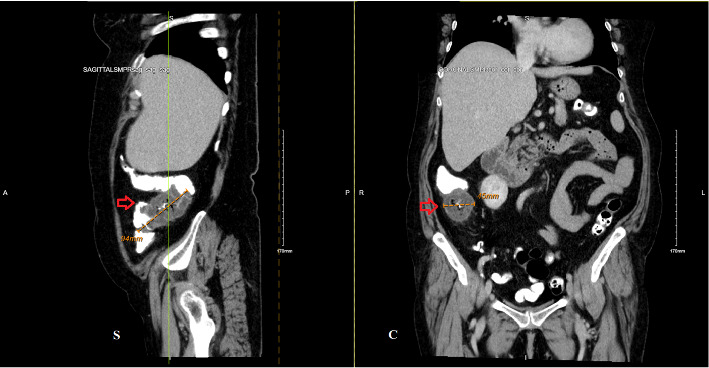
Contrast-enhanced abdominal computed tomography demonstrates an annular mass within the proximal ascending colon (red arrows in sagittal (S) and coronal (C) sections), measuring 94 mm in length and 45 mm in diameter with overhanging edges and subtle stranding of the pericolonic fat. Multiple subcentimeter adjacent lymph nodes are noted, not depicted on the provided images.
